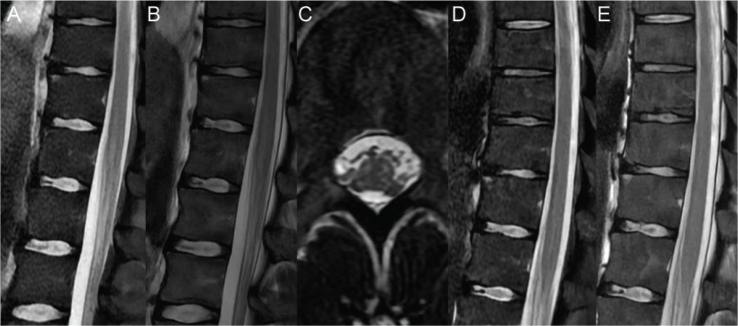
MRI imaging (A. First sagittal Short Tau Inversion Recovery (STIR), B. First magnetic resonance imaging (MRI) sagittal T2, C. First MRI axial T2, D. Second MRI sagittal STIR, E. second MRI sagittal T2).
